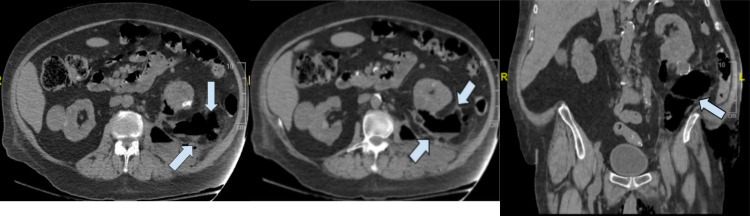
CT cross sectional images The left and middle panels are transverse sections and the right panel is a coronal section showing air forming infection starting in the left retroperitoneum extending to the left scrotum (arrows).
